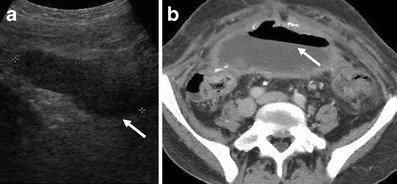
Anterior pelvic abscess seen on ultrasound (a) as complex fluid collection (*arrow*) containing debris and axial CT (b) showing an air-fluid level (*arrow*) in a patient on long-standing CAPD with peritoneal calcifications from encapsulating sclerosing peritonitis
